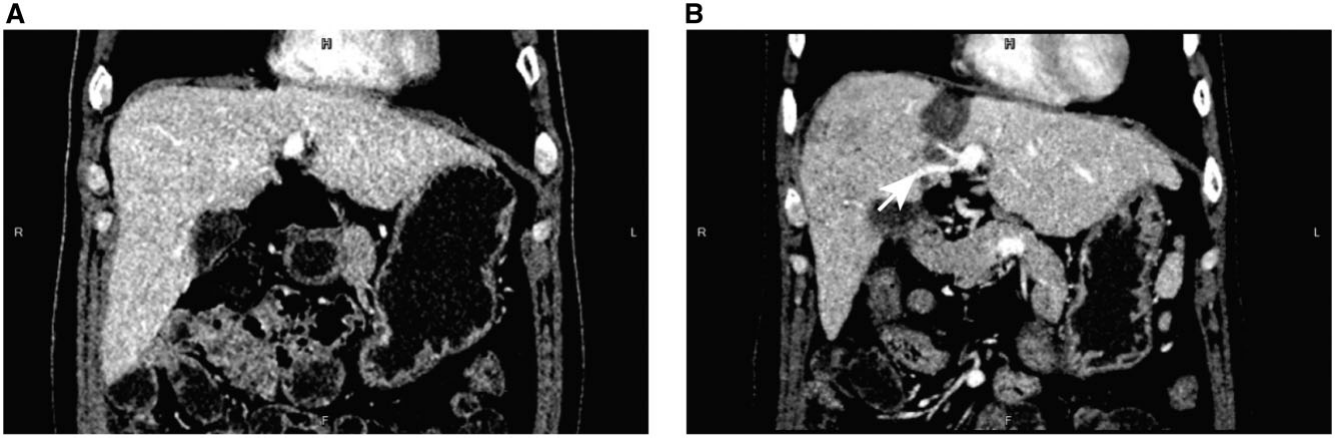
Contrast-enhanced computed tomography images show intrahepatic portoportal venous collateral vascular formation after liver partition and portal vein right branch ligation. (A) Before liver partition and portal vein right branch ligation. (B) Fourteen days after liver partition and portal vein right branch ligation (arrowhead: intrahepatic portoportal venous collateral vascular).
